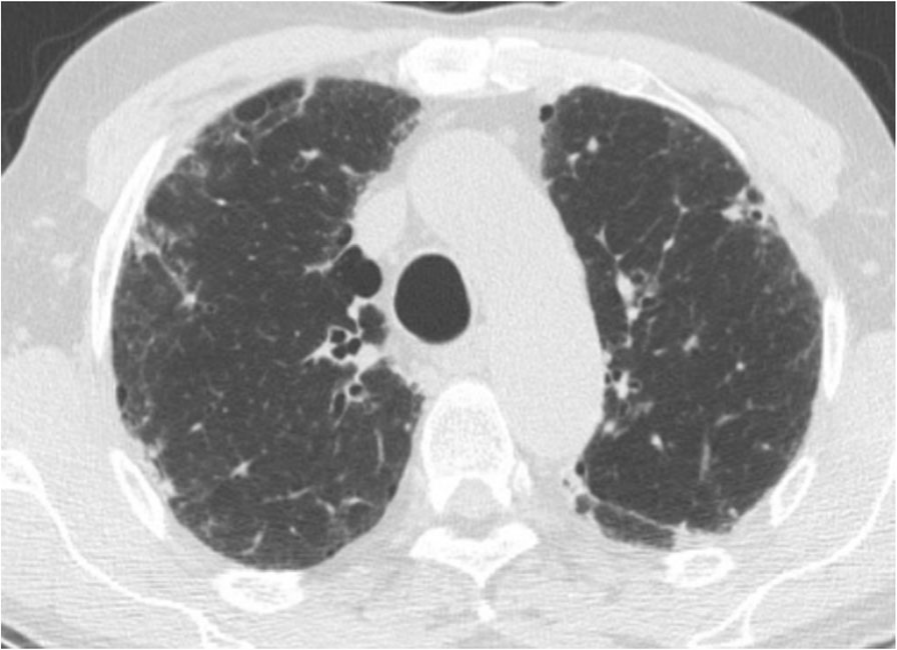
High resolution CT scan of one patient affected by PPFE, showing severe pleural and sub-pleural thickening with moderate fibrotic changes in the marginal parenchyma
